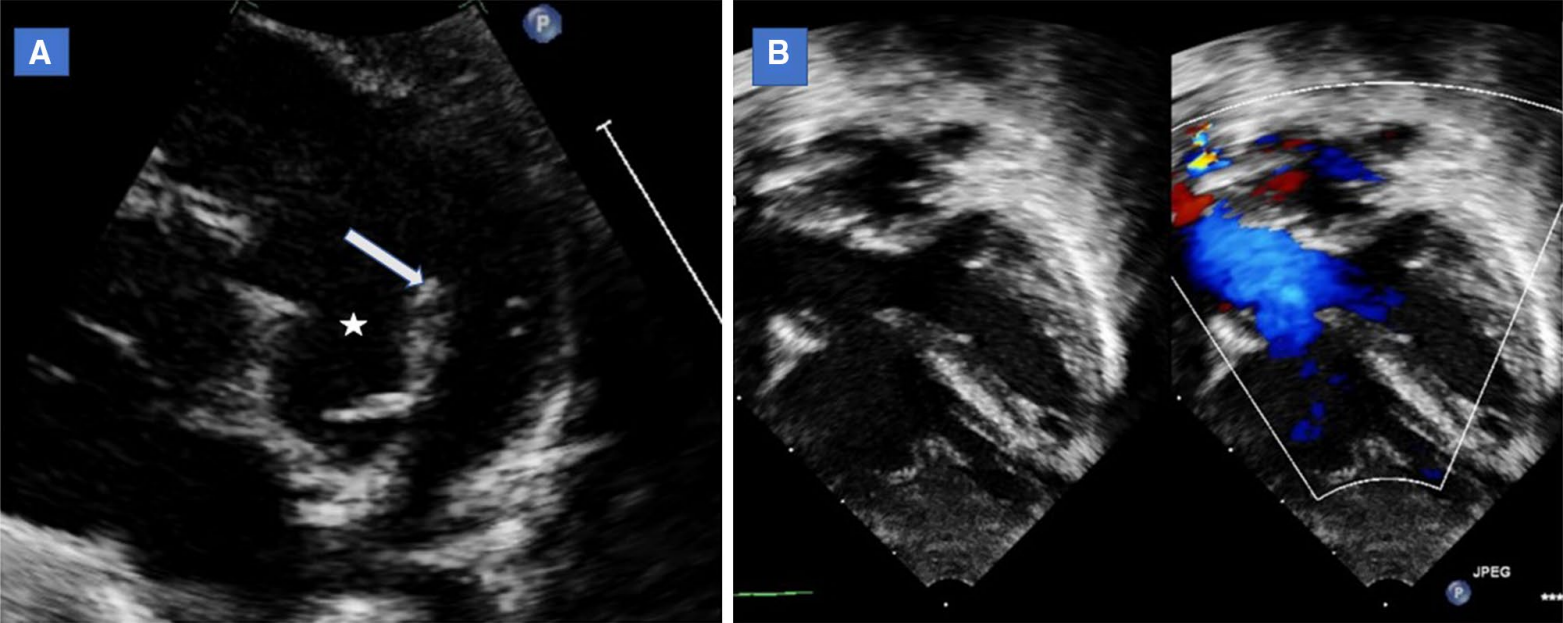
Echocardiographic images showing main features of tetralogy of Fallot. a Parasternal short-axis image showing anterior deviation of the conal septum (arrow) and resultant ventricular septal defect shown (asterisk). b. Apical five chamber echo view showing aortic override to the right ventricle and maligned ventricular septal defect
